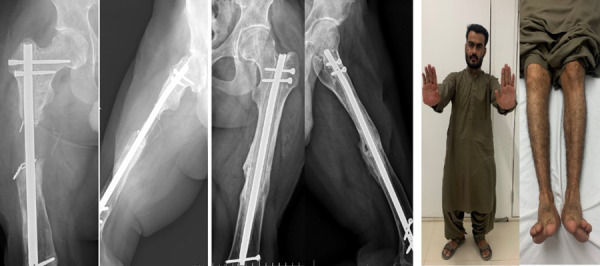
Follow-up images demonstrating successful outcome: right femur AP and lateral radiographs at one-year follow-up (left), left femur AP and lateral radiographs at two-and-a-half-year follow-up (middle), and corresponding clinical photograph showing functional ambulation (right), with maintained alignment and no evidence of recurrent infection.
